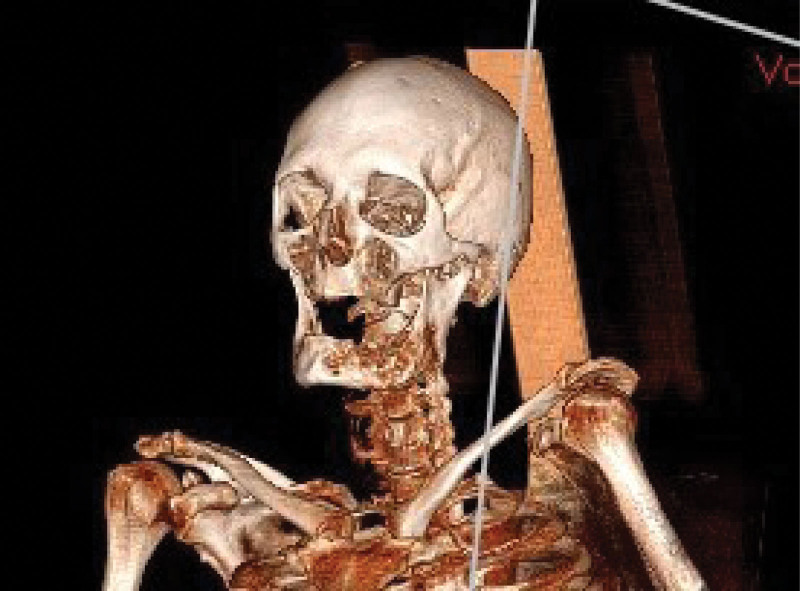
3D CT scan of jaw. CT = computed tomography.
